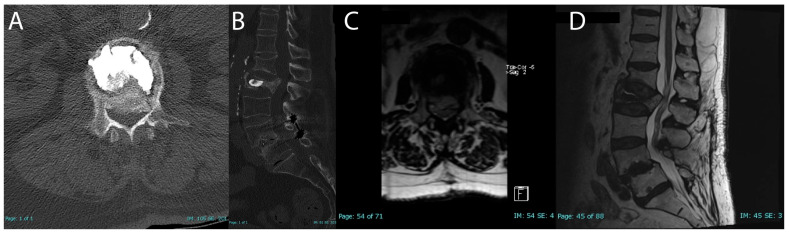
Follow-up complication for case 2 (A–D); one-month postoperative CT axial (A); CT sagittal (B); one-month postoperative MRI axial T2 (C); MRI sagittal T2 (D).
